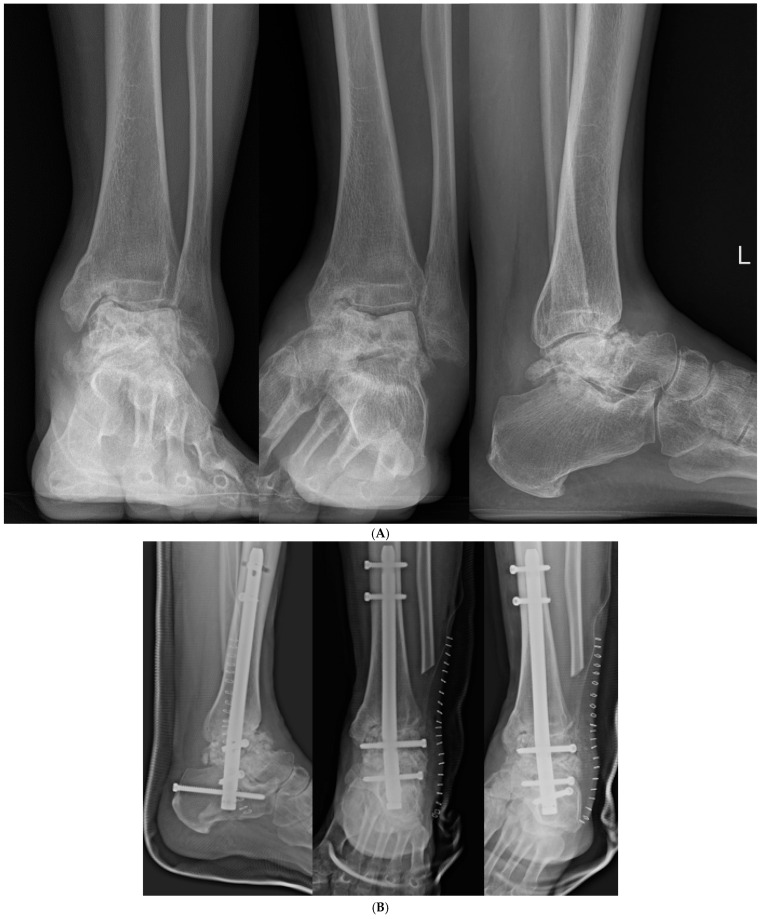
(A) Preoperative and (B) postoperative X-ray Findings.
